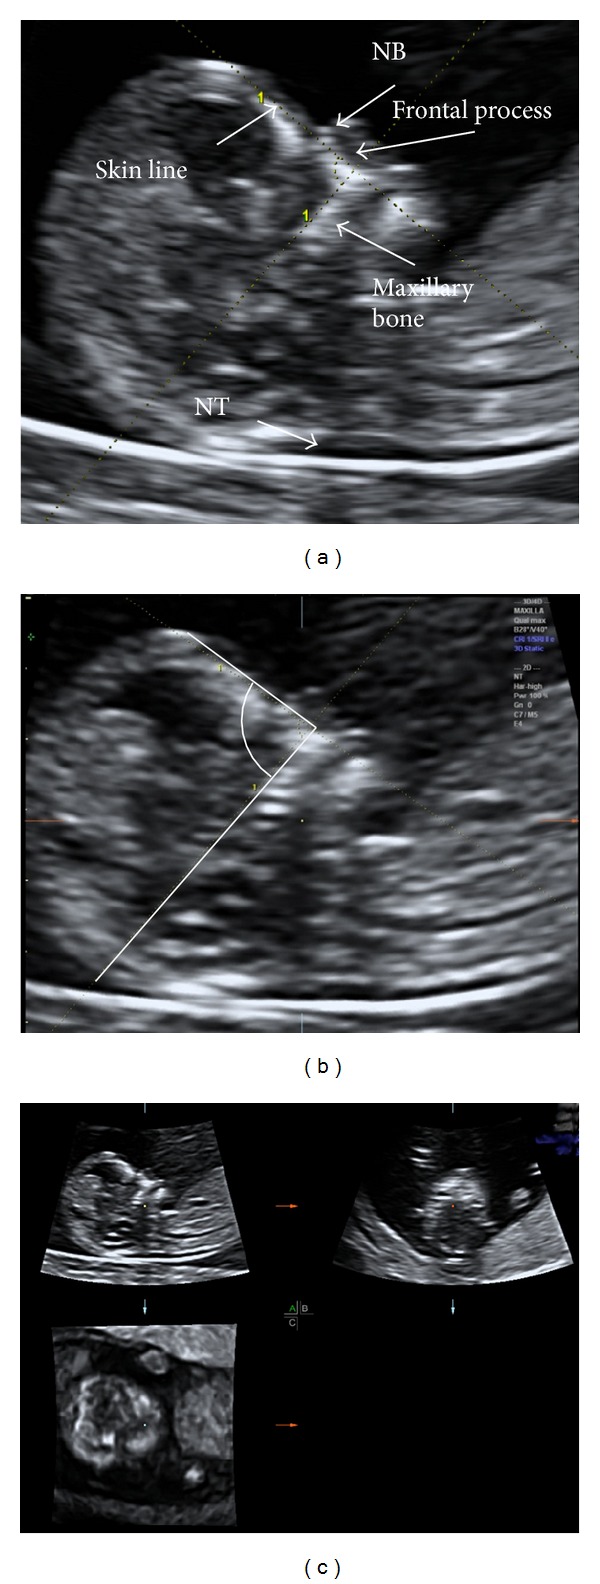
(a) 2D fetal profile with sonographic landmarks. (b) 3D reconstruction of the same fetus with caliper placement for FMF angle measurement. (c) 3D sectional planes of the same fetus.
